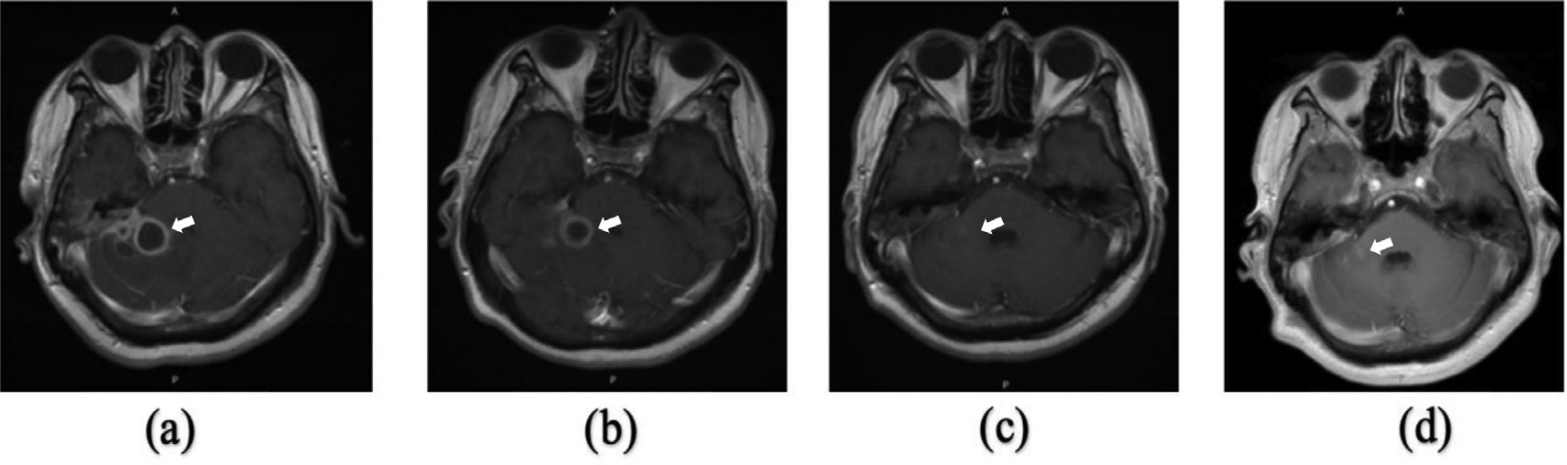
(a) MRI of the head with T1-weighted axial contrast before therapy. The lesion was seen pressing the right posterior aspect of the pons and the 4th ventricle medially, accompanied by leptomeningeal enhancement and right mastoiditis (arrow); (b) MRI of the head with T1-weighted axial contrast at week 5 showed the lesion appeared to be smaller; (c) T1-weighted axial contrast head MRI at week 22. The lesion showing contrast enhancement appeared to be shrinking; (d) 28th week T1-weighted axial contrast MRI of the head showing a relatively similar abscess size compared to week 22.
